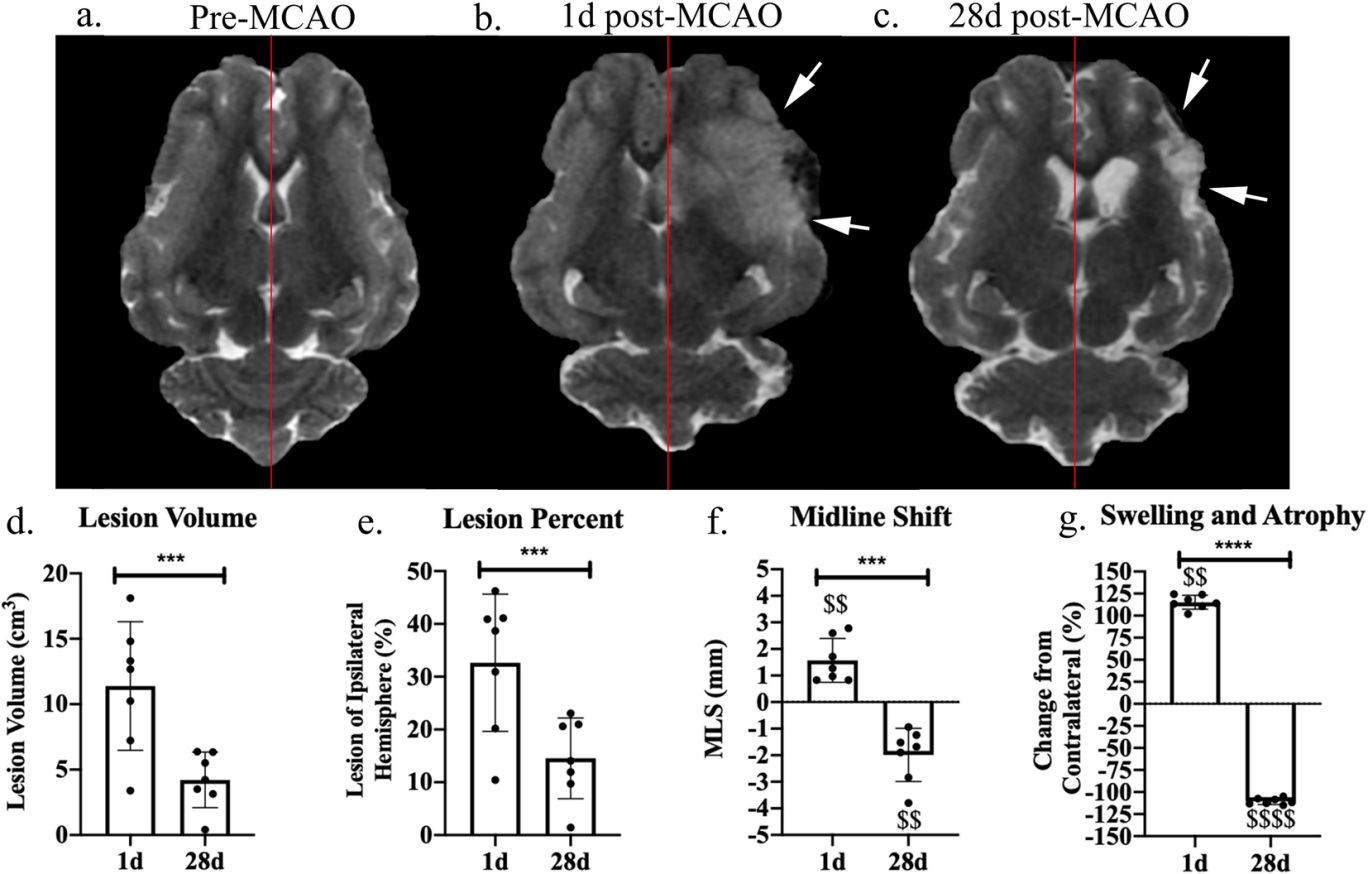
Canonical MRI measurements of MCAO induced ischemic stroke at 1d and 28d post-MCAO. T2W images prior to MCAO reveal homology between the hemispheres (a). At 1d (b) and 28d (c) post-MCAO, T2W images exhibited territorial hyperintense lesions indicated by white arrows and a shift from the natural midline (red lines indicate natural midline). Lesion volume (d), lesion volume as a percent of the ipsilateral hemisphere (e), midline shift (MLS) (f), and hemispheric changes (g) were quantified at 1d and 28d post-MCAO. * and  indicated statistically different between timepoints. $$ and $$$$ indicated statistically different from hypothetical normal.
